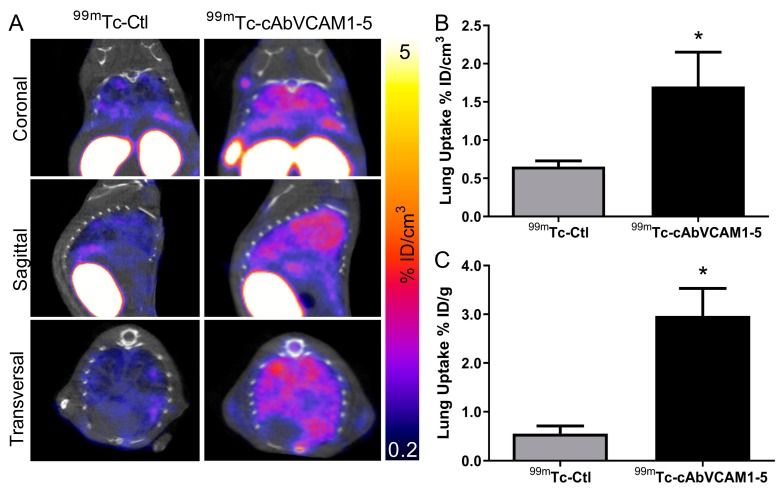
In vivo biodistribution of ^99m^Tc-cAbVCAM1-5 in a pulmonary experimental metastasis model. (A) Representative coronal and transversal views of fused SPECT/CT images of MDA-MB-231 lung metastases at 1 h after i.v. injection of ^99m^Tc-cAbVCAM1-5 or ^99m^Tc-Ctl. (B) In vivo quantification of ^99m^Tc-cAbVCAM1-5 and ^99m^Tc-Ctl lung uptake from SPECT images. (C) Ex vivo quantification of ^99m^Tc-cAbVCAM1-5 and ^99m^Tc-Ctl lung uptake from post-mortem biodistribution studies. Results were expressed as % ID/cm^3^ (imaging) and % ID/g (ex vivo) of tumor. Statistics: * *p* < 0.05 vs. ^99m^Tc-Ctl.
